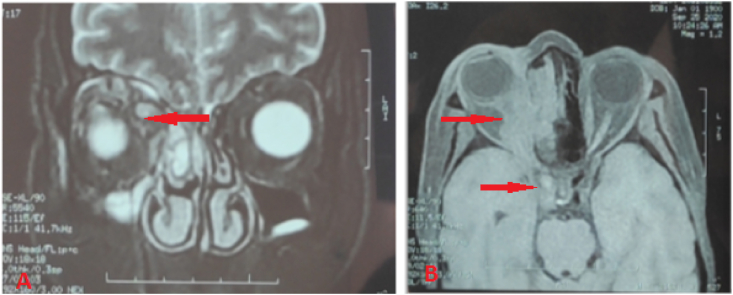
Magnetic resonance imaging (MRI) with improved contrast. (A) Coronal plane, periorbital swelling. (B) Distended superior ophthalmic vein on the right side, with a non-fatty density filling defect on the right side of the cavernous sinus.
